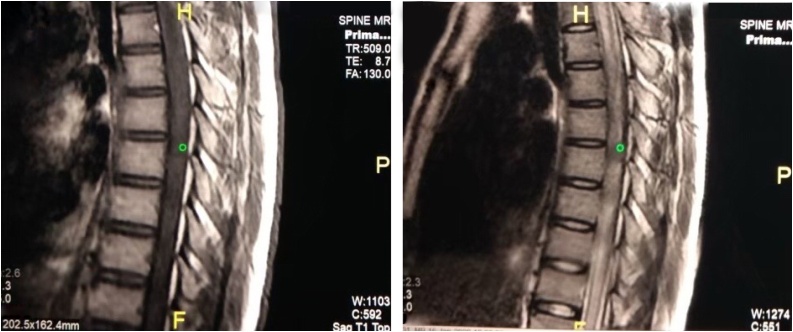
MRI sagittal view. Left: T1-weighted image showed intramedullary hypointense mass within T6. Right: T2-weighted image demonstrated low signal intensity in T6 level.
